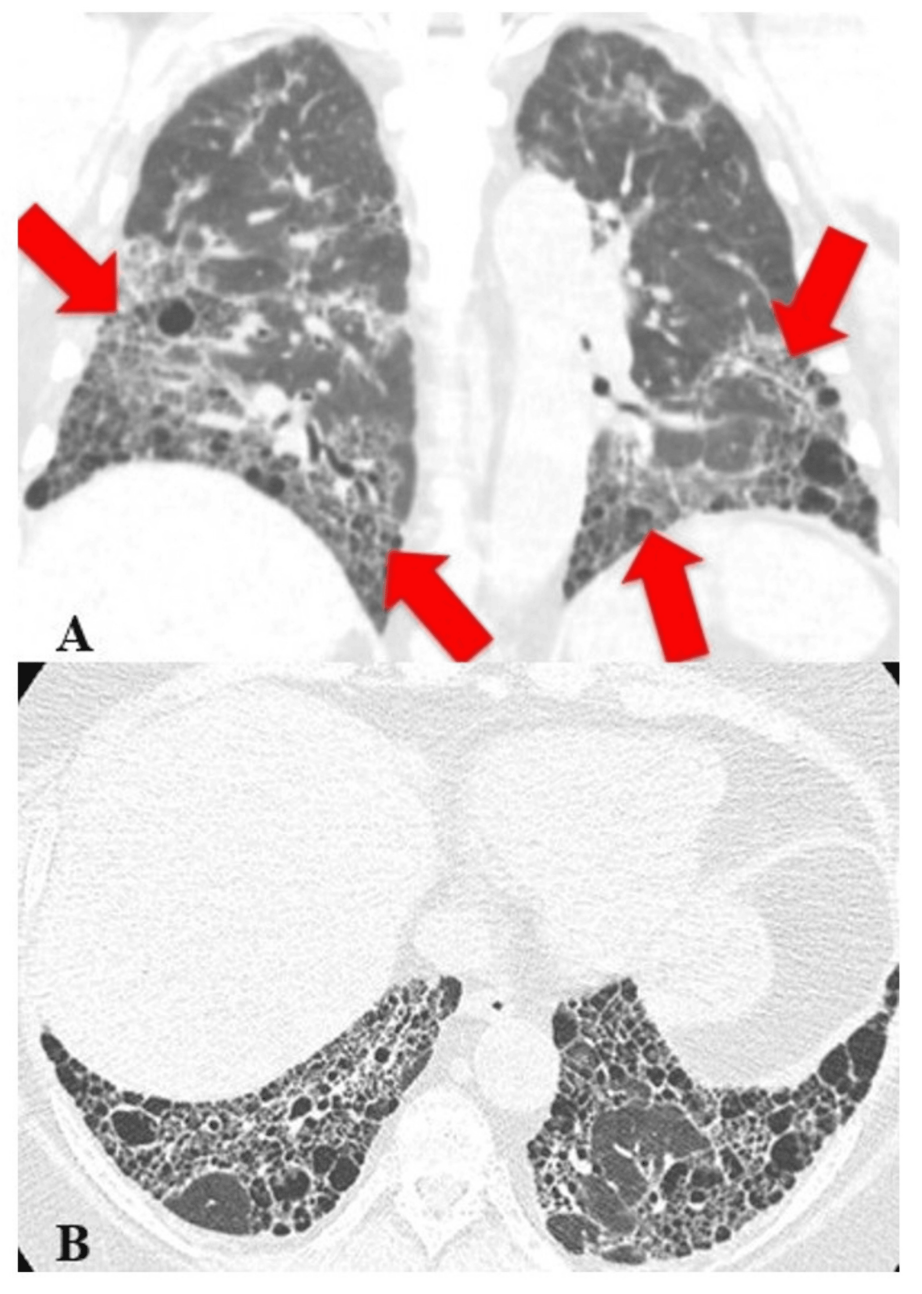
Moderate ILD with subpleural and basilar predominance (A) Axial CT image of the chest shows extensive confluent areas of reticular opacity in both lungs. (B) Coronal CT image highlights the bibasilar predominance of the involvement in this patient. ILD: interstitial lung disease, CT: computed tomography Image credit: Dr. Jordan Ditchek, MD (used with permission)
